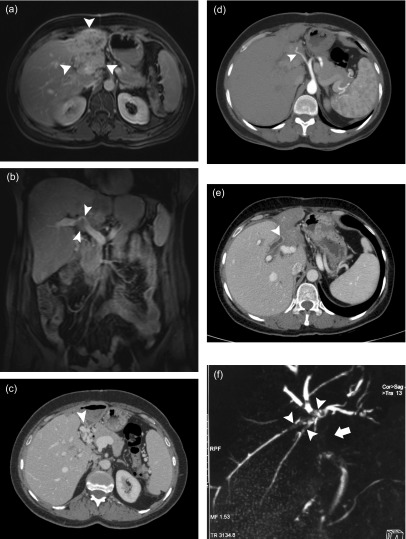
A 51-year-old female patient presented with back pain. (a) Abdominal MRI with contrast demonstrates a mass lesion (*arrowheads*) with indistinct borders, which completely fills the left lobe of the liver with concurrent atrophy and shows diffuse heterogeneous enhancement. (b) Postcontrast coronal MRI image demonstrates portal vein invasion and narrowing (*arrowheads*). (c) Many collateral veins (*arrowhead*) that developed at the hilus secondary to portal vein invasion are demonstrated by portal phase CT examination. (d) An arterial phase axial CT image reveals the hepatic artery wrapped by the mass (*arrowhead*). (e) Contrast-enhanced abdominal CT examination shows that the contrast-enhanced, thickened common bile duct is interrupted within the lesion (*arrowhead*). (f) Filling defects due to the bile ducts invaded by the mass (*arrowheads*) and the interruption of the common bile duct (*arrow*) are more clearly visualized by MRCP images. Cholangiocarcinoma was considered in the differential diagnosis; however, the core biopsy result was consistent with AE.
